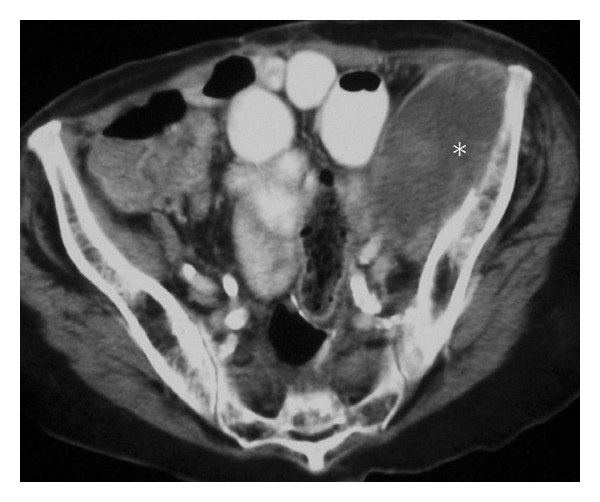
Post-radiation sarcoma. A 78-year-old female presenting with left upper thigh and hip pain. The patient had received radiotherapy to the pelvis 32 years previously, for an ovarian malignancy, and was later diagnosed with radiation-induced sarcoma. Axial contrast-enhanced CT identifies a large, heterogeneously enhancing mass along the inner surface of the left ilium (asterisk) with local bone destruction of the inner cortex.
